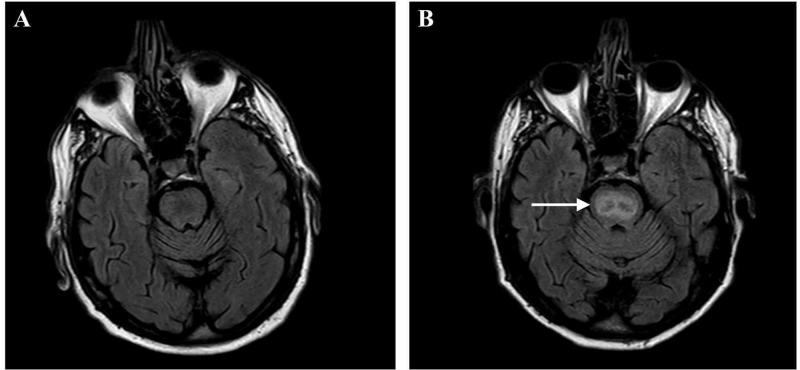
T2-weighted axial brain MRI of our patient (A) The first MRI, made during the first admission four days after the correction of hyponatremia, in which no significant abnormalities were observed. (B) The second MRI, made more than three weeks after the first MRI towards the end of the second admission, in which a clearly increased signal intensity is visible in the pons (denoted by →), characteristic for an osmotic demyelination syndrome.
